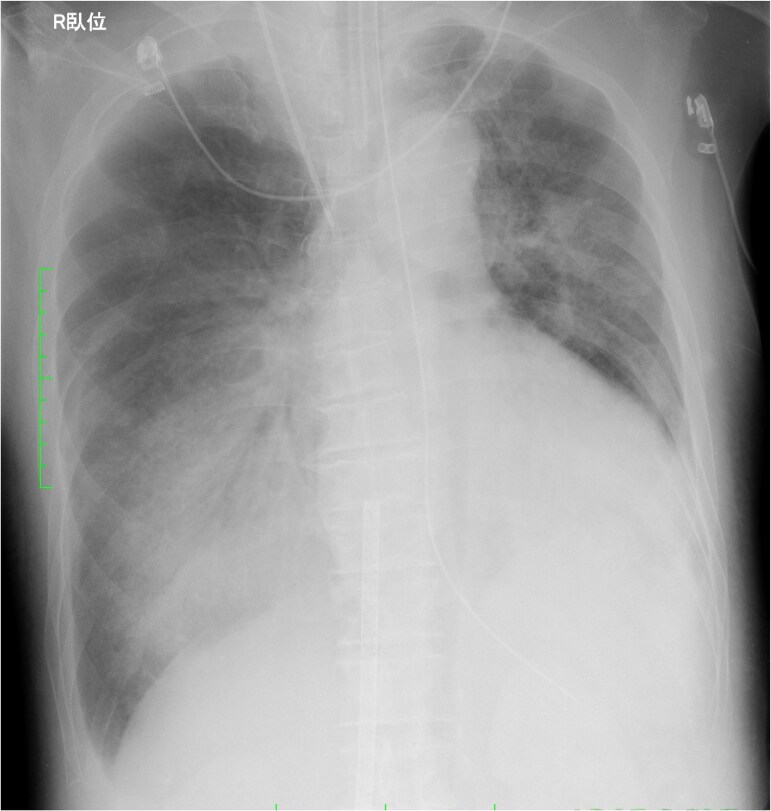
Following prone positioning, chest radiograph findings improved with an increase in air content.
